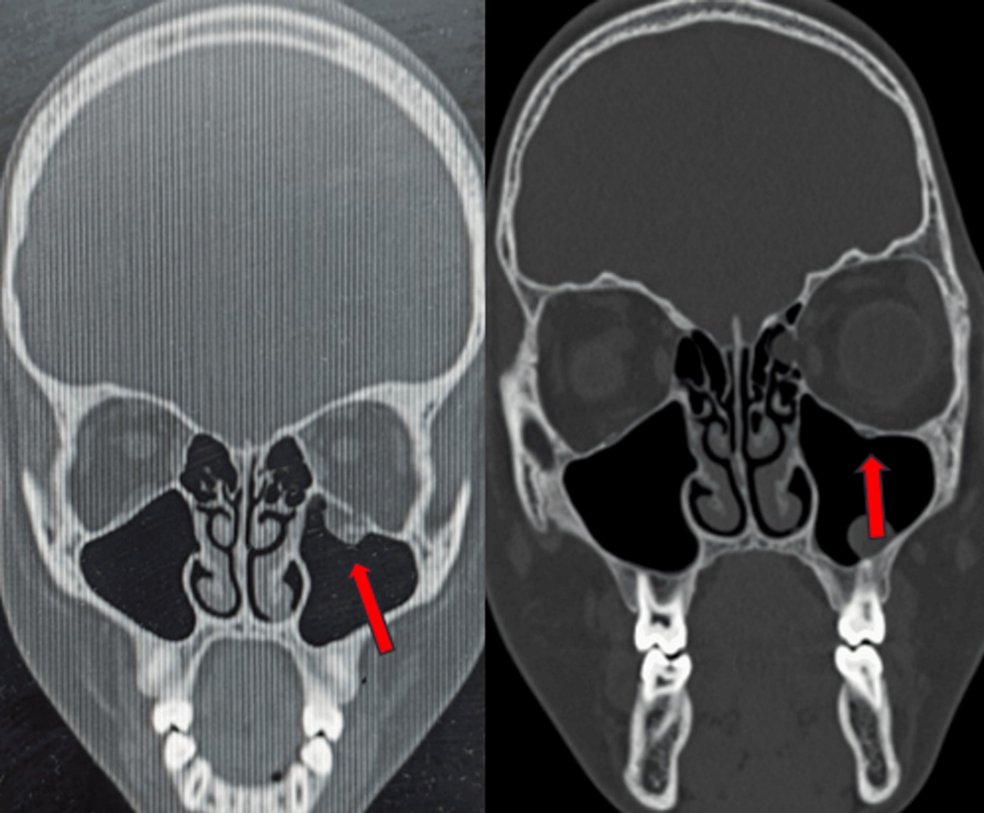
Case one: the coronal section of NCCT face shows pre and post, orbital floor fracture repair by iliac crest bone graft NCCT - non-contrast computed tomography
